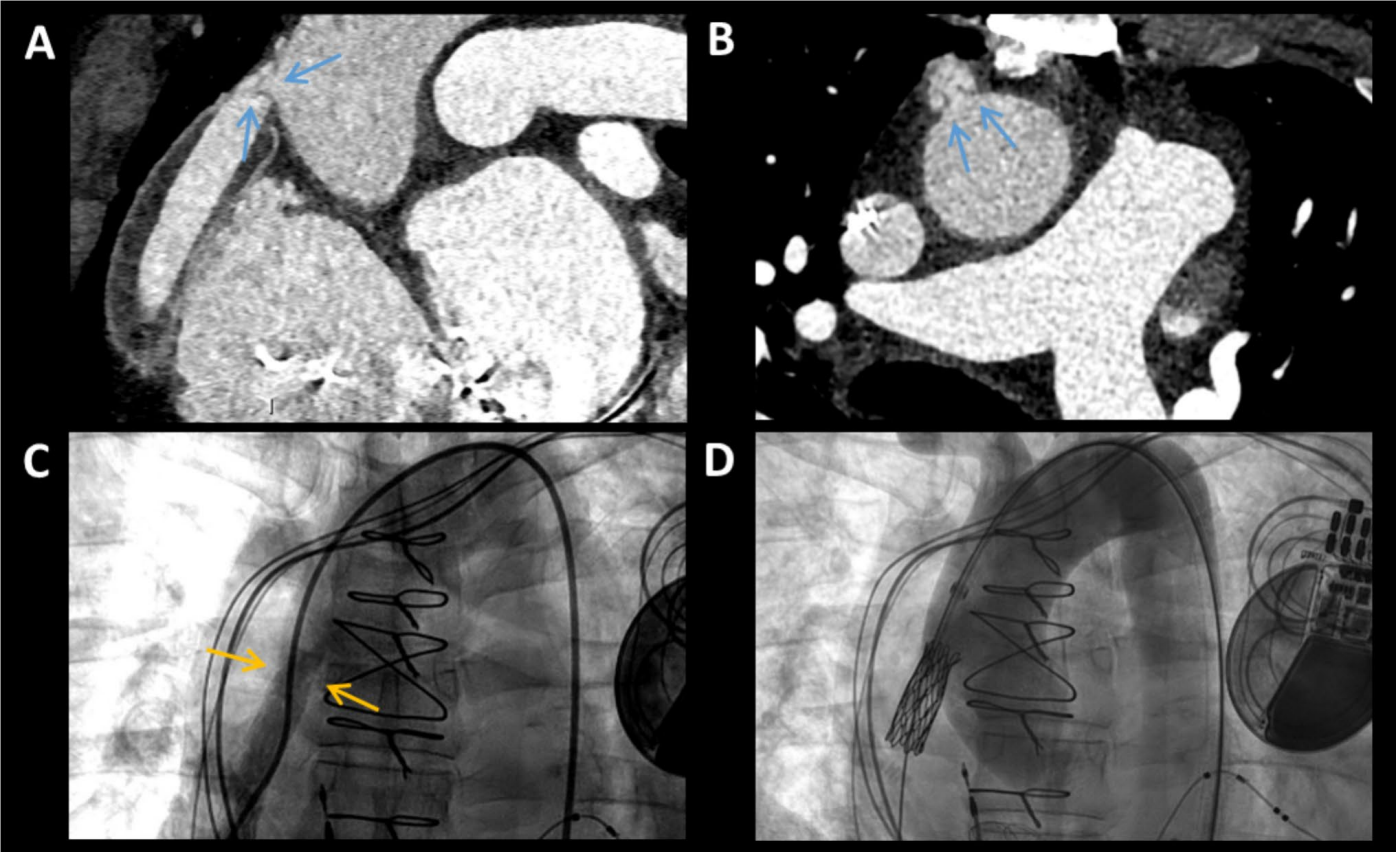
Obstruction of the Outflow Graft of Left Ventricular Assist Device. Cardiac computed tomography (CCT) in a longitudinal (A) and axial (B) plane shows a weblike thrombus *(blue arrows)* in the outflow graft of a left ventricular assist device (LVAD). Invasive angiography (C) demonstrated narrowing (*yellow arrows*) at the level of obstruction and the patient underwent stenting (D)
